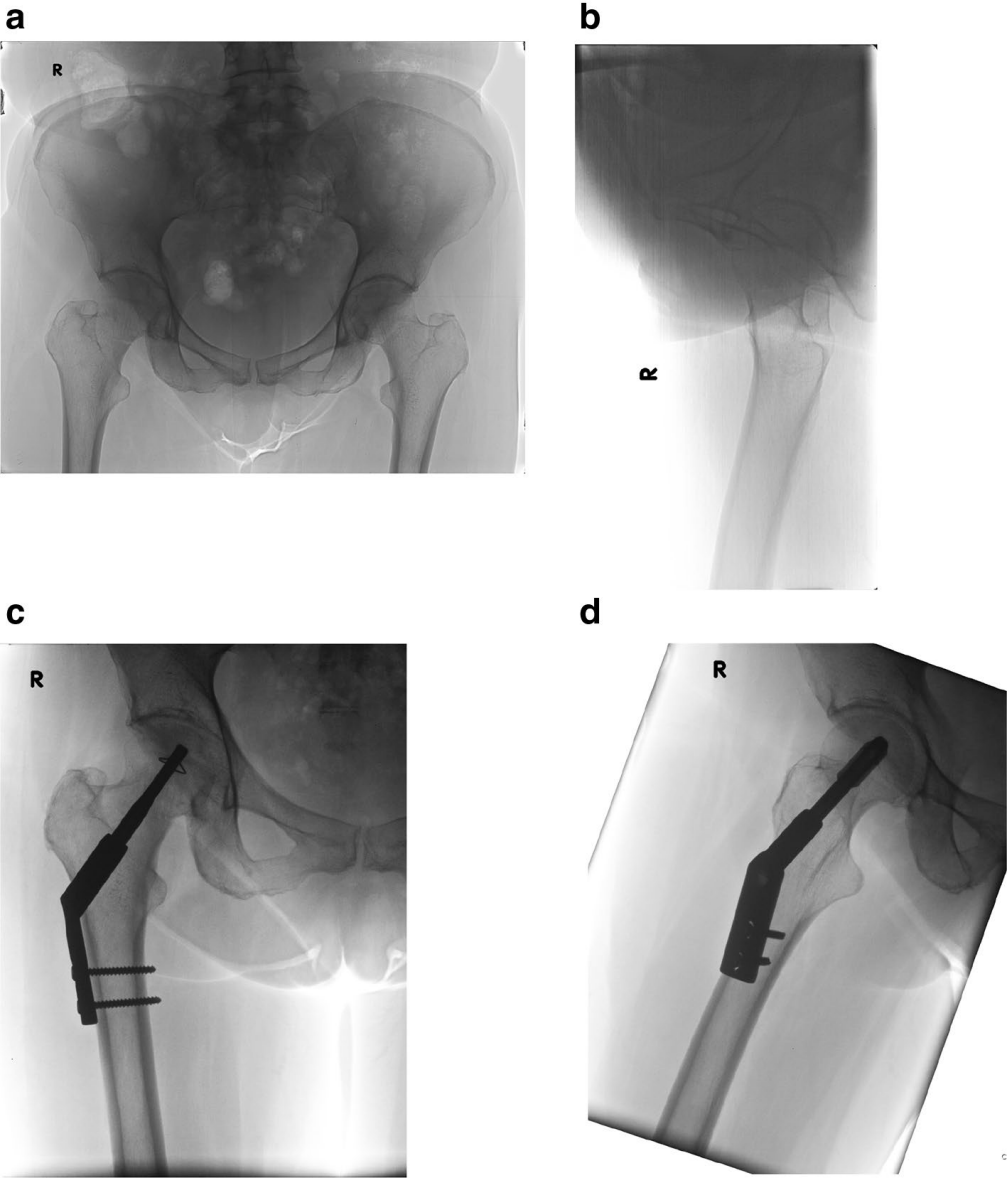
a, b AP en lateral X-ray of undisplaced femoral neck fracture of the right hip, c, d AP and lateral X-ray of undisplaced femoral neck fracture of the right hip after DLBP fixation
